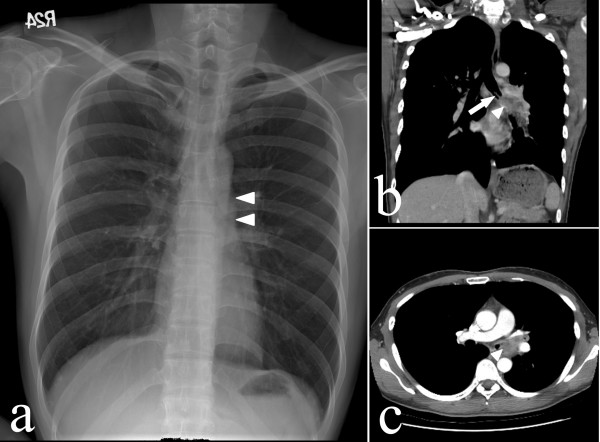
Posterior-anterior chest radiograph (Panel a) shows an ill-defined opacity around the left hilum (arrowheads). Chest computed tomography (Panel b and c) shows soft tissue density (arrowheads) within the left mainstem bronchus with a needle-shaped object (arrow) protruding from it.
